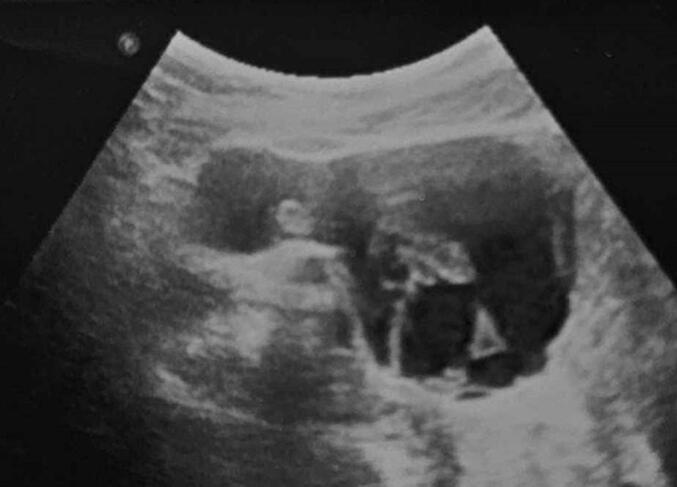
Ultrasonography view showing a simple 5 cm unilocular ovarian cyst with hyperechoic content with an enlarged right ovary.
